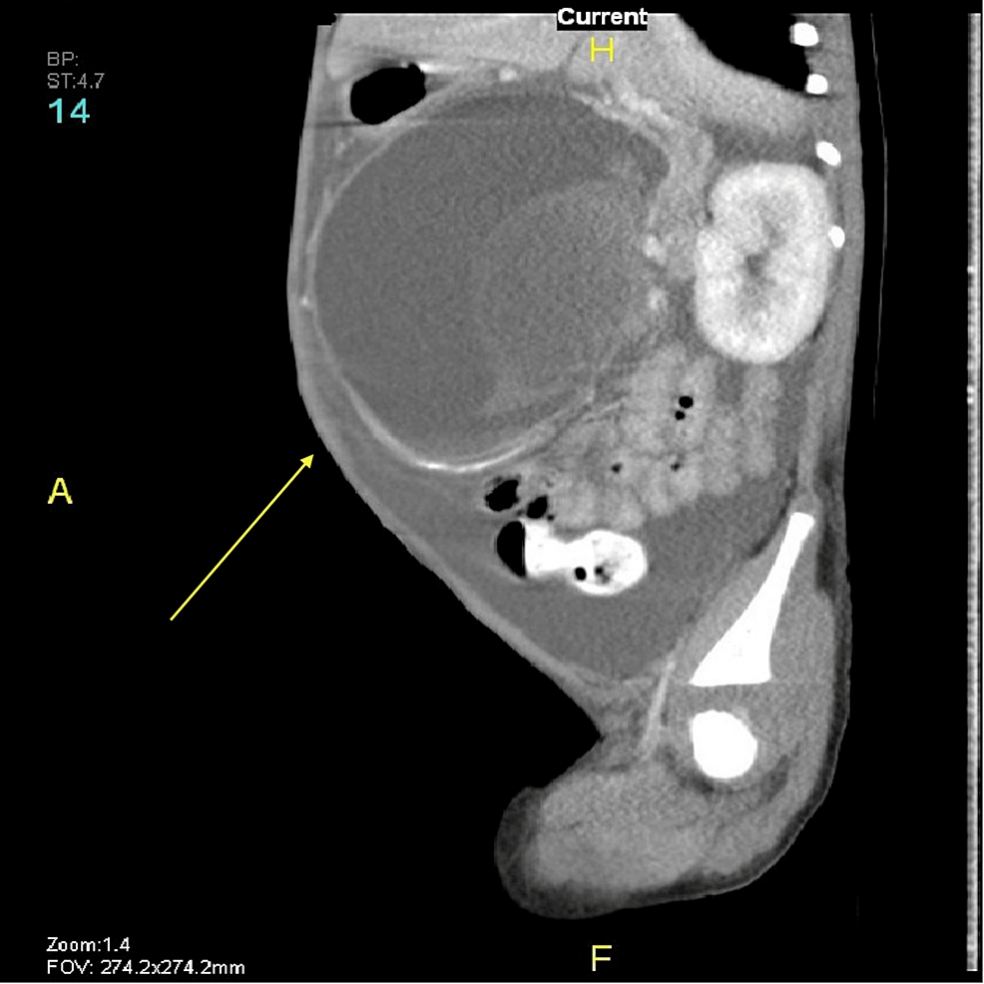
Abdominal CT scan showing pseudocyst in body and tail of the pancreas surrounded by fluid after follow-up (arrow) CT: computed tomography
